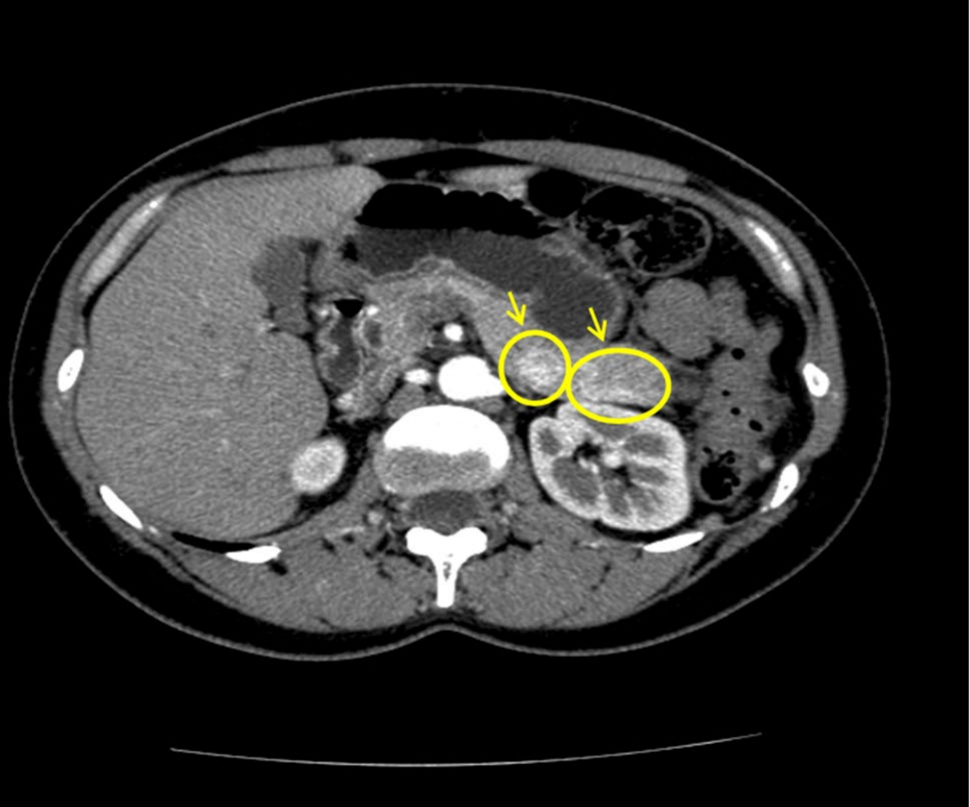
CT scan performed in 2012 showing two lesions in the body of the pancreas of 16 mm and 8 mm, respectively, with contrast enhancement features typical of insulinoma (marked with yellow arrows and circles)
